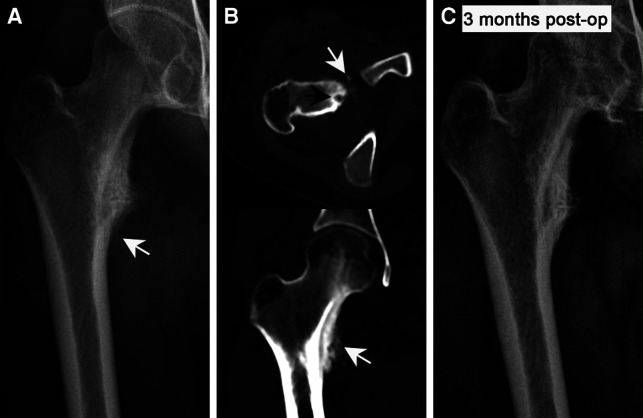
The preoperative and postoperative imaging characteristics of OO in a lesser trochanter. (A) The radiography shows the periosteal reaction caused by the nidus but the nidus is not visible. (B) Thin-layer CT accurately displays the nidus and the surrounding hyperplastic bone. (C) Postoperative radiographs show that the reaction bone was completely absorbed and the bone morphology was restored to normal (The white arrow shows the nidus, black arrows indicate reactive hyperplastic bone).
